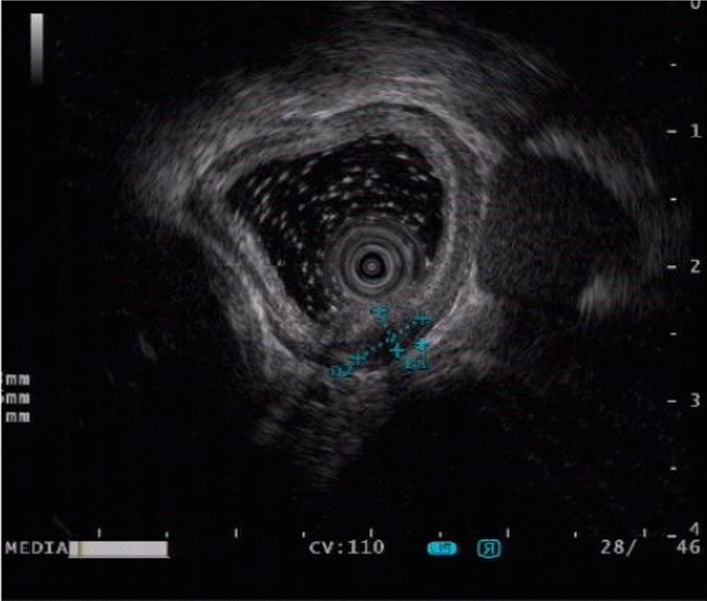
The ultrasound revealed an oval hypoechoic esophageal mass with homogeneous internal echo, originating from the muscularis propria, with a maximum cross section of 13 mm × 6 mm
